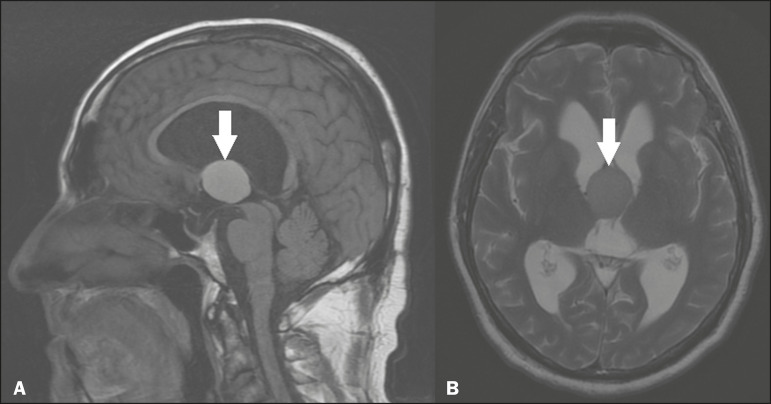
Colloid cyst. Sagittal T1-weighted MRI (A) and axial T2-weighted MRI (B), showing a cyst-like formation in the anterosuperior portion of the third ventricle, with a signal that was hyperintense in the T1-weighted sequence and hypointense in the T2-weighted sequence (arrows). Note the hydrocephalus.
